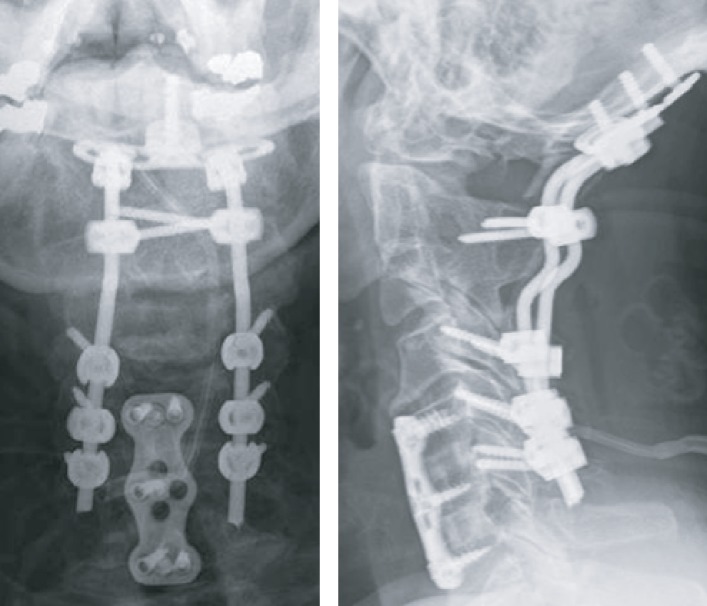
Case 1: postoperative X-ray. Occipito-C6 PSIF.
